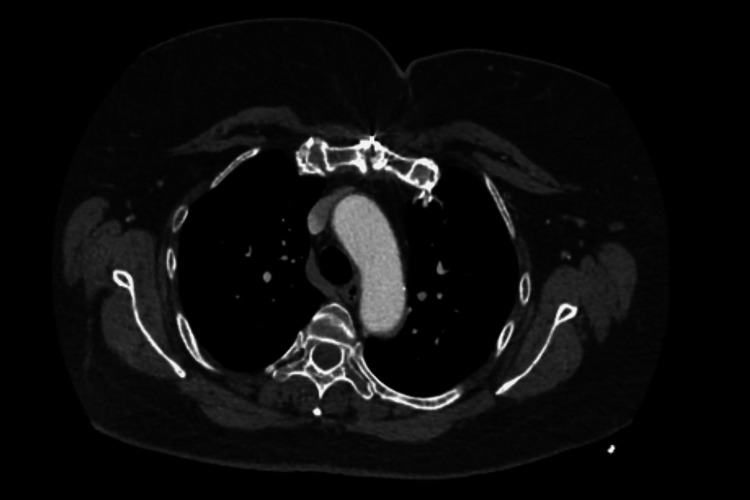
Follow-up CTA/aortogram three months following discharge showing total resolution of aortic arch thrombus. CTA: computed tomography angiogram
